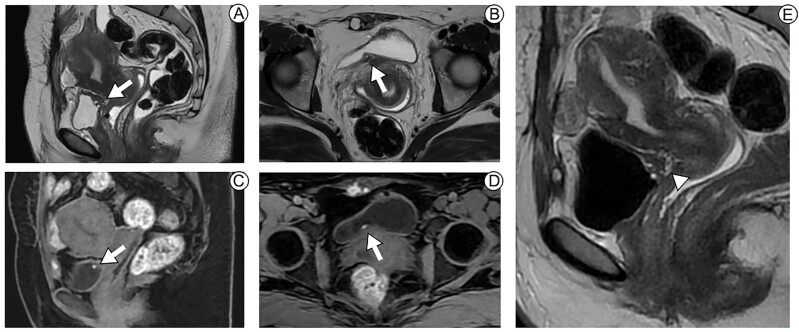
MRI shows DIE at the posterior aspect of the bladder (arrows), in the trigone closer to the right ureteral ostium. MRI sequences are T2-weighted at images A, E (sagittal plane) and B (axial plane). MRI sequences are T1-weighted at images C (sagittal plane) and D (axial plane). Late MRI sequence was performed (E) to allow bladder distension (note the bladder hypointense signal intensity related to Gadolinium contrast media), improving the lesion - ureteral distance measurement. There is muscle and mucosal infiltration.
